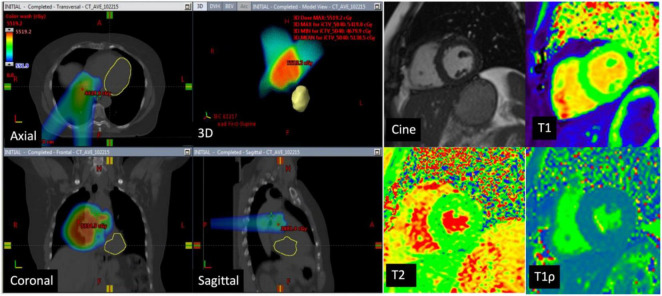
CT images in a 73-year-old man with lung cancer—Axial, coronal, sagittal, and 3D CT images with color-washed dose distribution. The heart is depicted in yellow and is not in the planned radiation field. The patient received a total of 50.4 Gy of which the mean dose to the left ventricle was 0.02 Gy. Cardiac magnetic resonance (CMR) images at baseline with normal LVEF (65%), normal native T1 mapping (1,043 ms), normal T2 mapping (47 ms), and elevated T1ρ at 78 ms. Follow-up CMR in 6 months showed no significant difference in native T1 and T2 values and the T1ρ values remained elevated.
